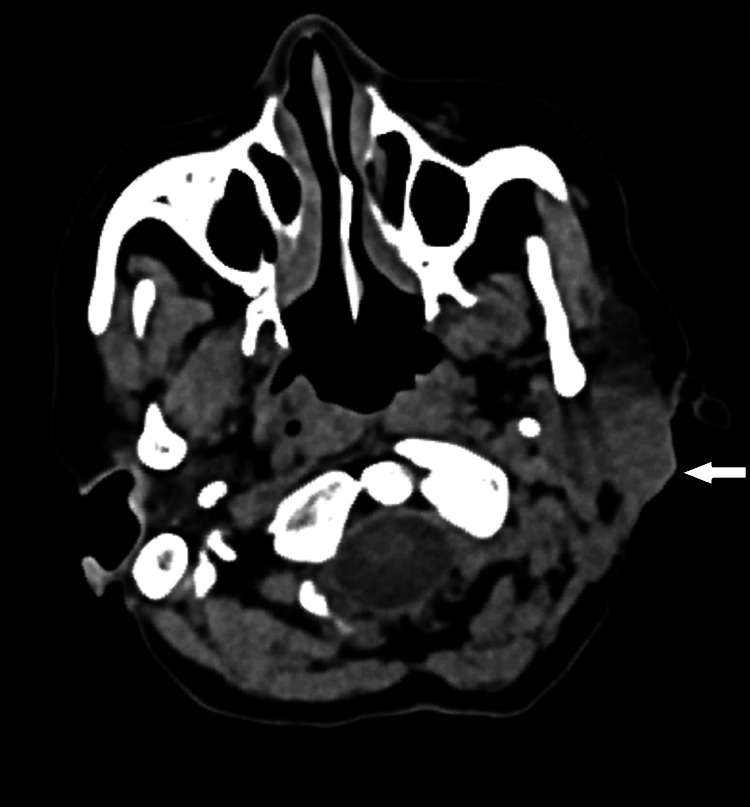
CT image showing the deep and superficial lobes of the left parotid gland with the bulge in the superficial lobe (arrow)
